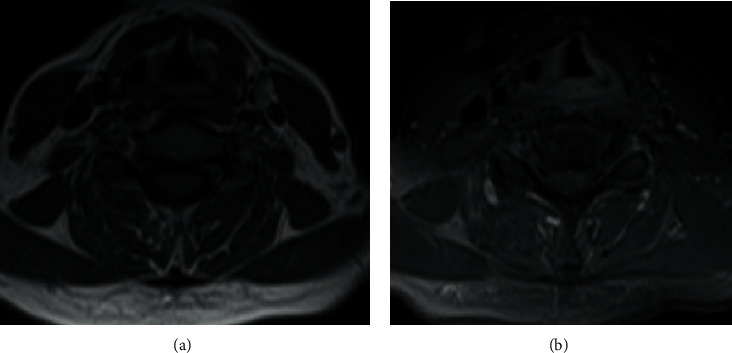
Axial MRI images demonstrating a nonenhancing lesion with fatty marrow changes arising from the left thyroid cartilage. (a) T1 without contrast. (b) T1 fat saturation with contrast.
